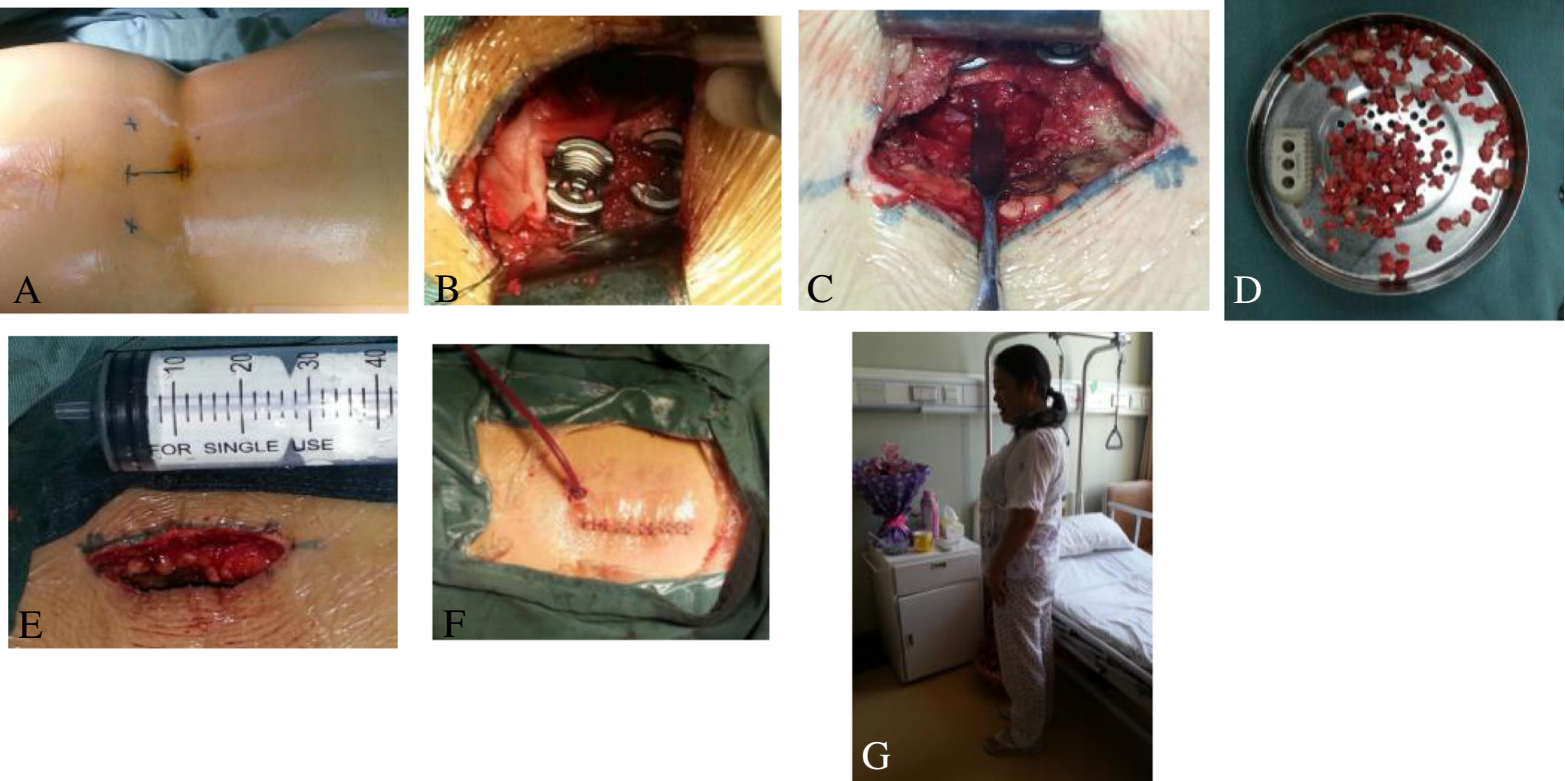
a–g Posterior lumbar interbody fusion using a unilateral cage with unilateral pedicle screws. a The patients were placed in the prone position under general anaesthesia. b The lamina and posterior joints of the symptomatic side were exposed, and a transpedicular screw system was placed under X-ray guidance. c Unilateral facetectomy and hemilaminectomy were performed on the symptomatic side, and the symptomatic nerve root was detected and decompressed carefully. d Autogenous morselised bone obtained from the laminectomy and facetectomy was inserted into the cage and disc space. e, f The length of the incision was not greater than 3 cm. g Three days after surgery, the patients could get out of bed and walk
